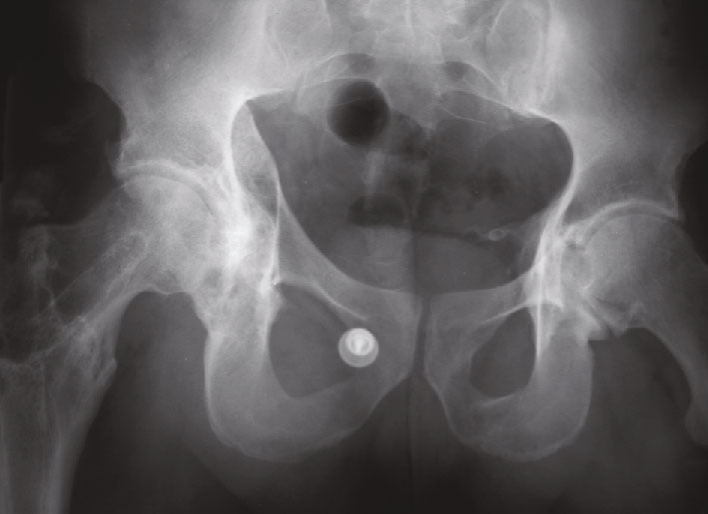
Follow-up X-ray at 2 years postsurgery.
